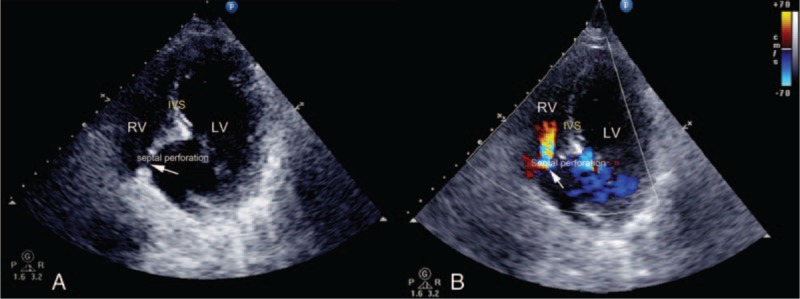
(A) Nonstandard left ventricular short-axis section at the apical level showing a ventricular septal defect (arrow). The site of septal perforation was the apex, adjacent to the septum–free wall junction. (B) Color Doppler shows a left-to-right shunt at the level of the ventricular septal perforation.
